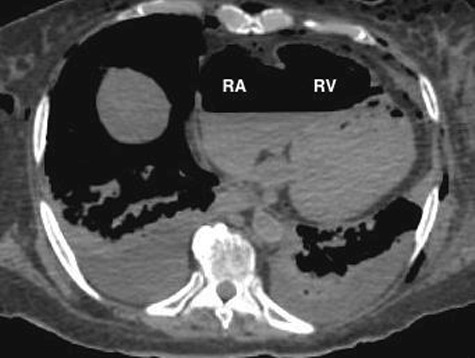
Axial CT of chest demonstrates large amount of air within the right atrium (RA) and right ventricle (RV) (lung window).
